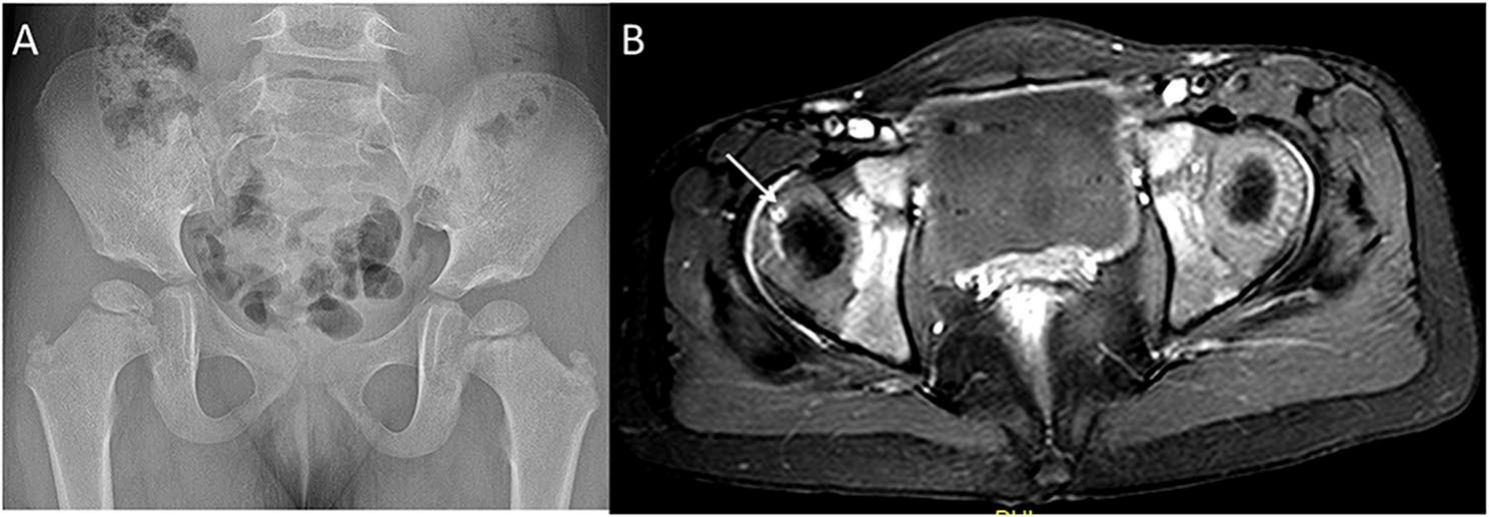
A 3-year-old girl with right LCPD. (A) The pelvic radiograph. (B) Axial enhanced MR image. The arrow shows the thickened cartilaginous vessels of the right FH; the cartilaginous vessels are fewer than those on the opposite side. MR = magnetic resonance, FH = femoral head, LCPD = Legg–Calvé–Perthes disease
